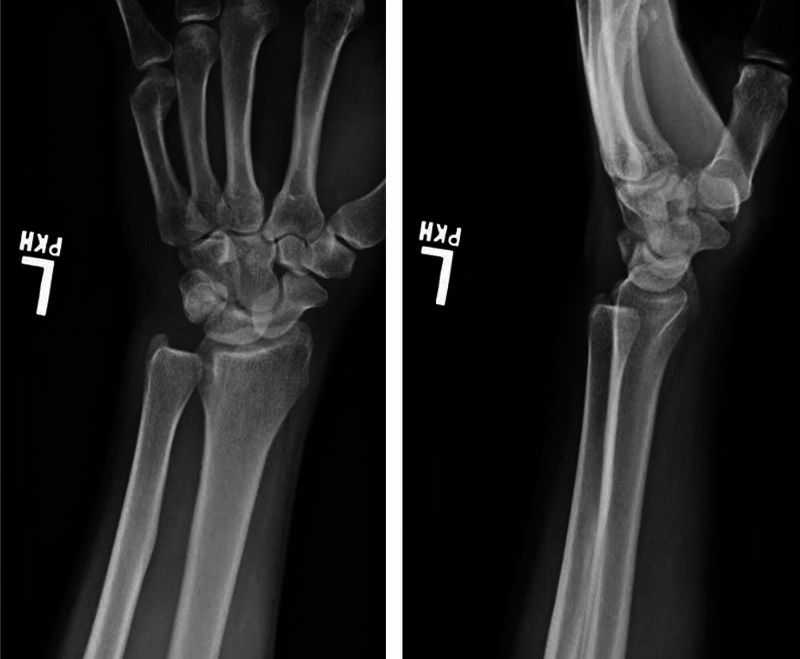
Posteroanterior (left) and lateral (right) radiographs taken at follow-up demonstrating maintenance of reduction without recurrence of volar ulnar dislocation
